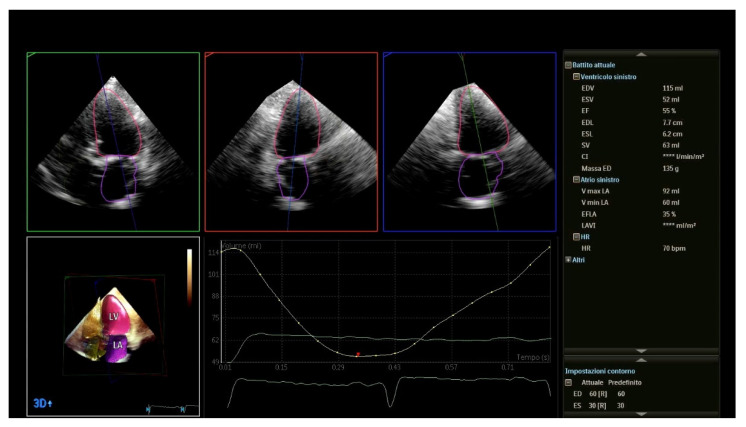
Three-dimensional measurements (LV volumes) obtained from automated DHM in the same patient. This advanced echocardiography allows for accurate, automated measurements of chamber volumes and function, providing larger and more accurate LV volumes with good agreement with cardiac magnetic resonance when analyzed using the default settings of the boundary detection sliders (end-diastolic default position = 60/60; end-systolic default position = 30/30). The calculated Rvol = SVLV – SVForward = 63 − 40 mL = 20 mL. EROA = Rvol/VTIRegJet = 20/186 = 0.10 cm^2^. LV: left ventricular; DHM: Dynamic Heart Model, Philips Healthcare, Andover, MA, USA; Rvol: regurgitant volume; SVLV: stroke volume ejected by the left ventricle; SVForward: forward stroke volume. 2D: bi-dimensional echocardiography; PISA: proximal isovelocity surface area; 3D: three-dimensional echocardiography; MR: mitral regurgitation; EROA: effective regurgitant orifice area.
